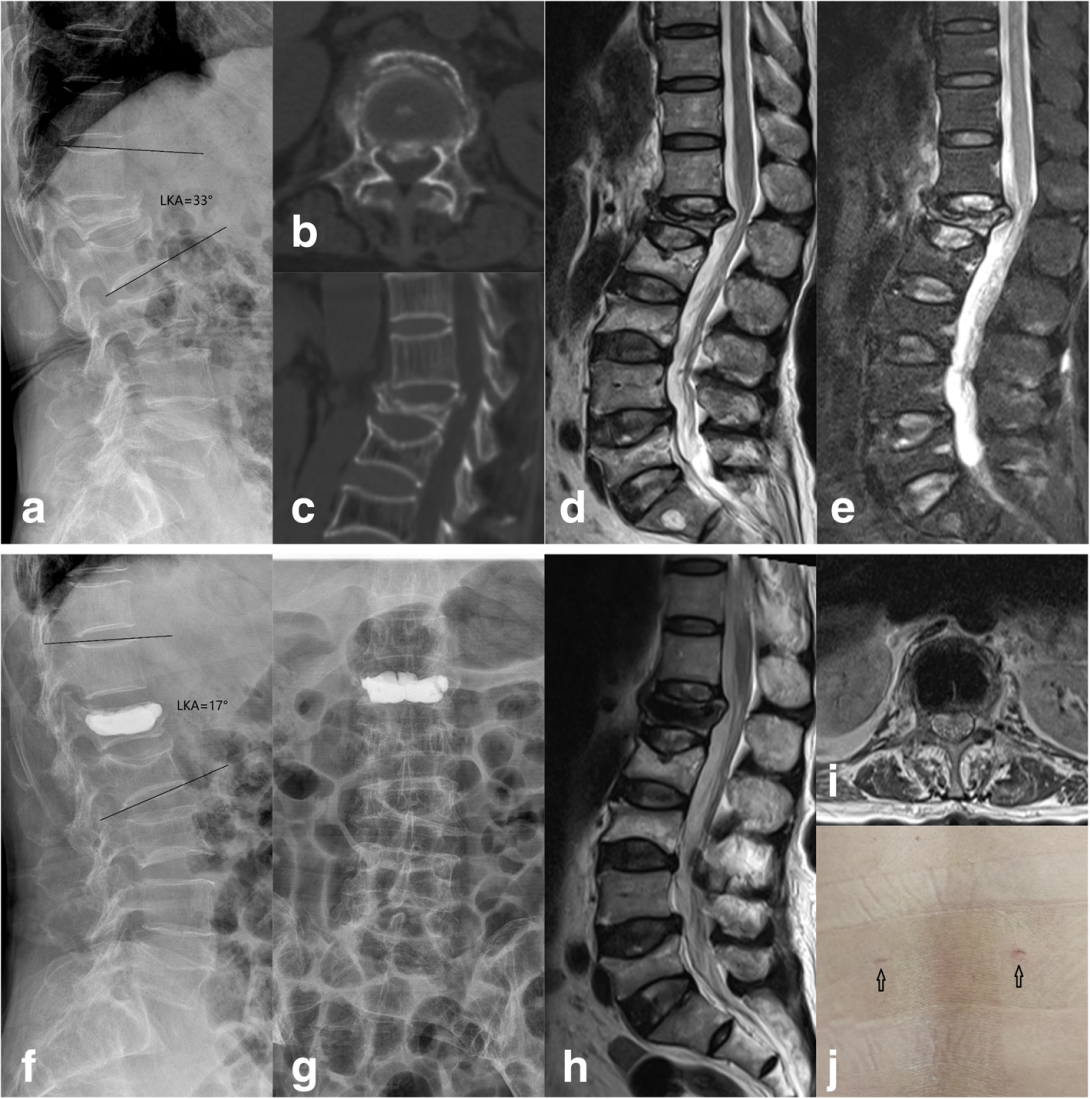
A 69-year-old woman suffered L1 vsOVCF with spinal canal compromise treated with percutaneous kyphoplasty. a The pre-operative kyphotic angle was 33°. b, c Pre-operative CT showed retropulsion of the posterior wall fragment into the spinal canal. d, e The pre-operative MRI displayed high signal intensity in the vertebral body in T2-weighted and short tau inversion recovery (STIR) images. f, g The post-operative X-ray films showed cement distributed well and did not leak. The kyphotic angle was 17°. h, i The post-operative MRI showed reduction of the retropulsed bone fragment from the spinal canal. j The minimal incisions of PKP (black arrows)
